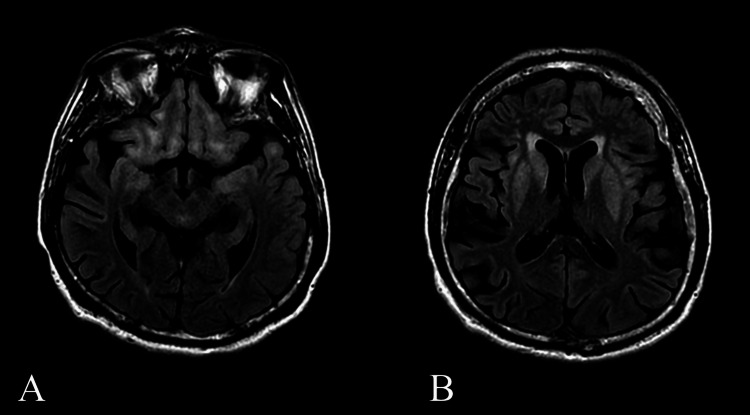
MRI findings in July 2018 A, B: axial T2W-FLAIR MRI taken in July 2018 depicting bilaterally symmetric hyperintensities in the (A) orbital frontal lobes, medial temporal lobes, (B) subinsular cortical areas, and basal ganglia with temporal cortical atrophy T2W: T2-weighted; FLAIR: fluid-attenuated inversion recovery; MRI: magnetic resonance imaging
